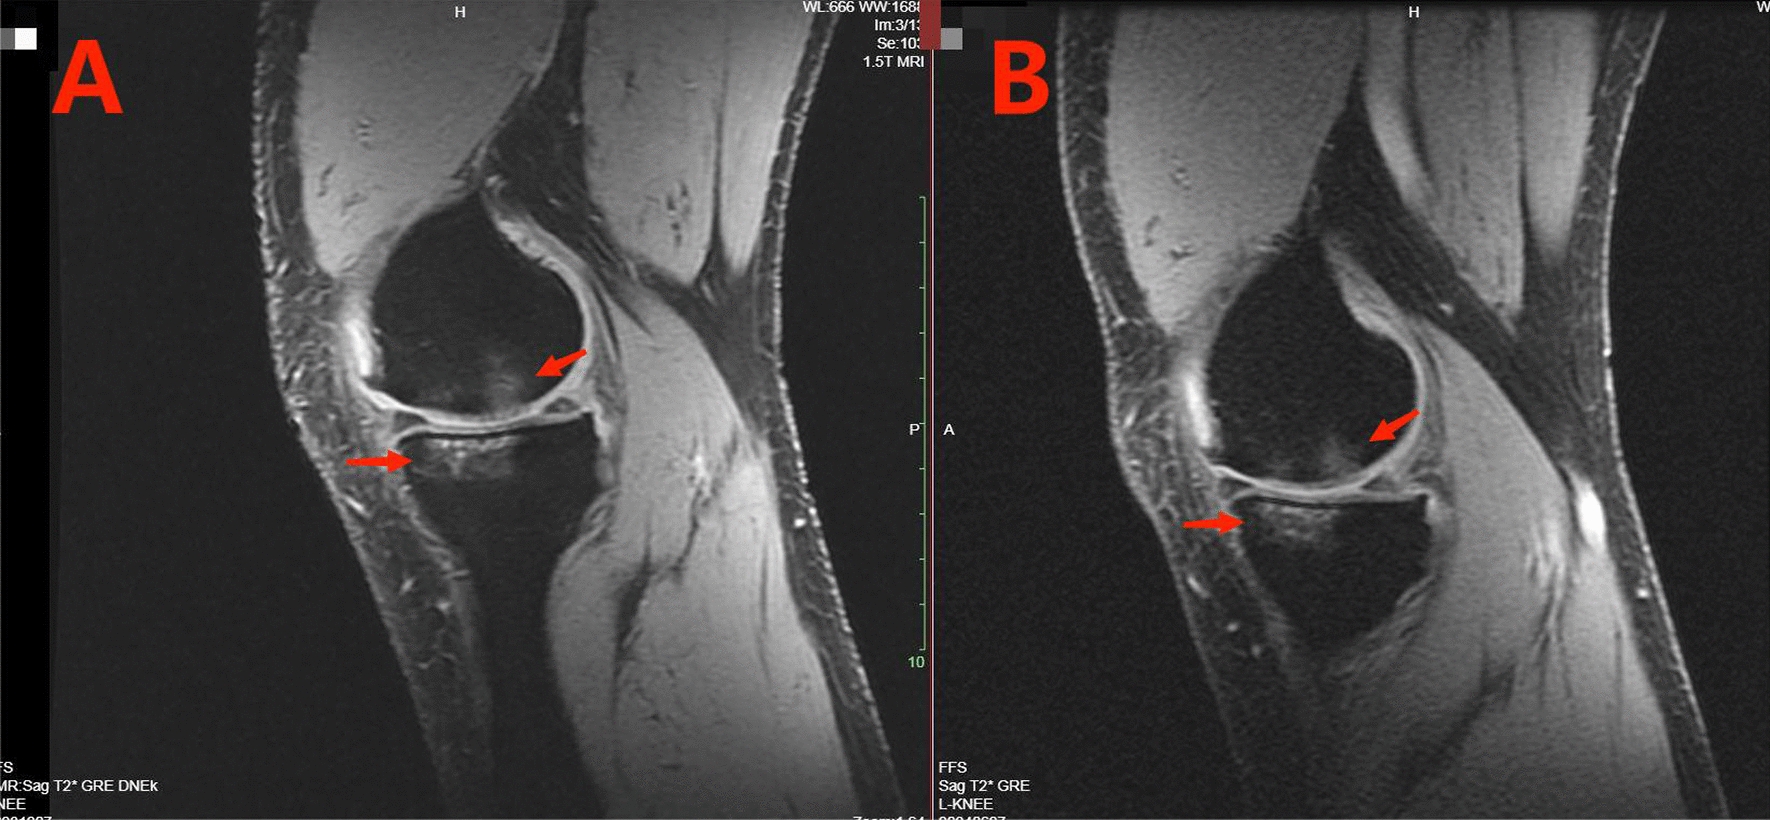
Pre-treatment (A) and 6 months post-treatment (B) MRI sagittal T2-weighted images of a 53-year-old male patient with osteoarthritis of the left knee. Subchondral bone marrow edema (indicated by arrows in the MRI image) was reduced at 6 months post-treatment compared to pre-treatment
